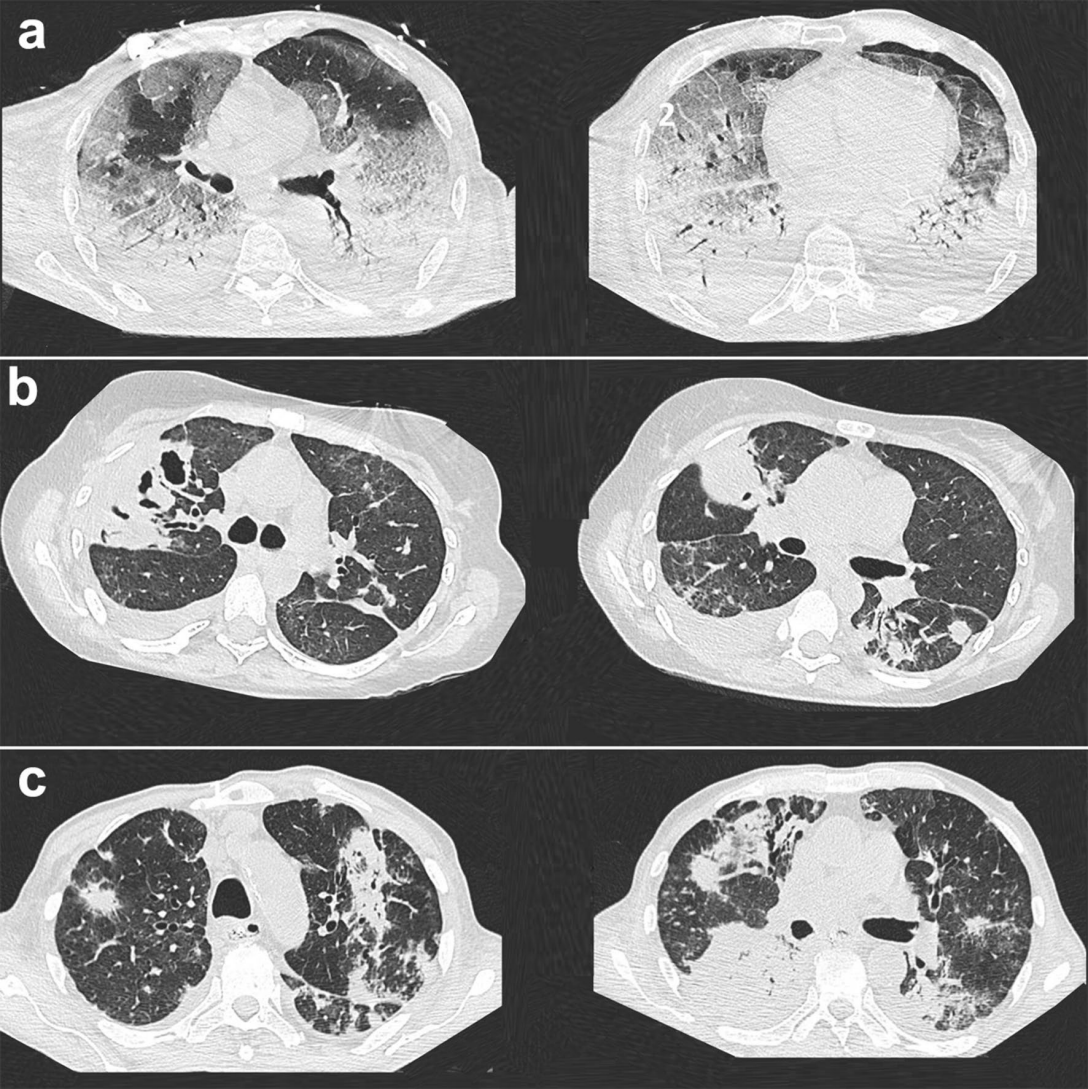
Chest CT scan images in patients with ARDS and one or more respiratory tract culture positive for *Aspergillus* spp., categorized as having putative invasive pulmonary aspergillosis (IPA) or *Aspergillus* colonization [16]. CT scan slices depicted a ARDS-typical bilateral basal consolidations, together with ground-glass opacities (*left panel*) and left anterior pneumothorax (*right panel*) in a patient categorized as having putative IPA; b
